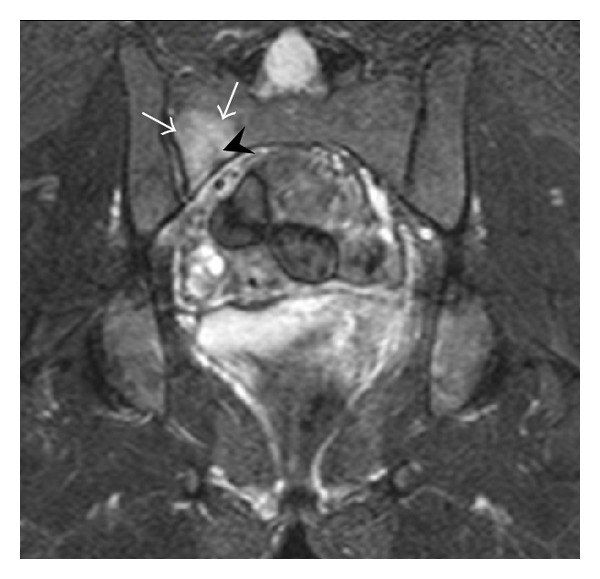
Right sacral alar insufficiency fracture in a 29-year-old woman with a 9-year history of corticosteroid therapy for systemic lupus erythematous. Conventional radiographs showed normal appearance (not shown). Coronal inversion recovery MRI shows an area of hyperintensity in the right sacral ala (white arrows), centered on a linear hypointensity corresponding to the fracture line (black arrowhead).
